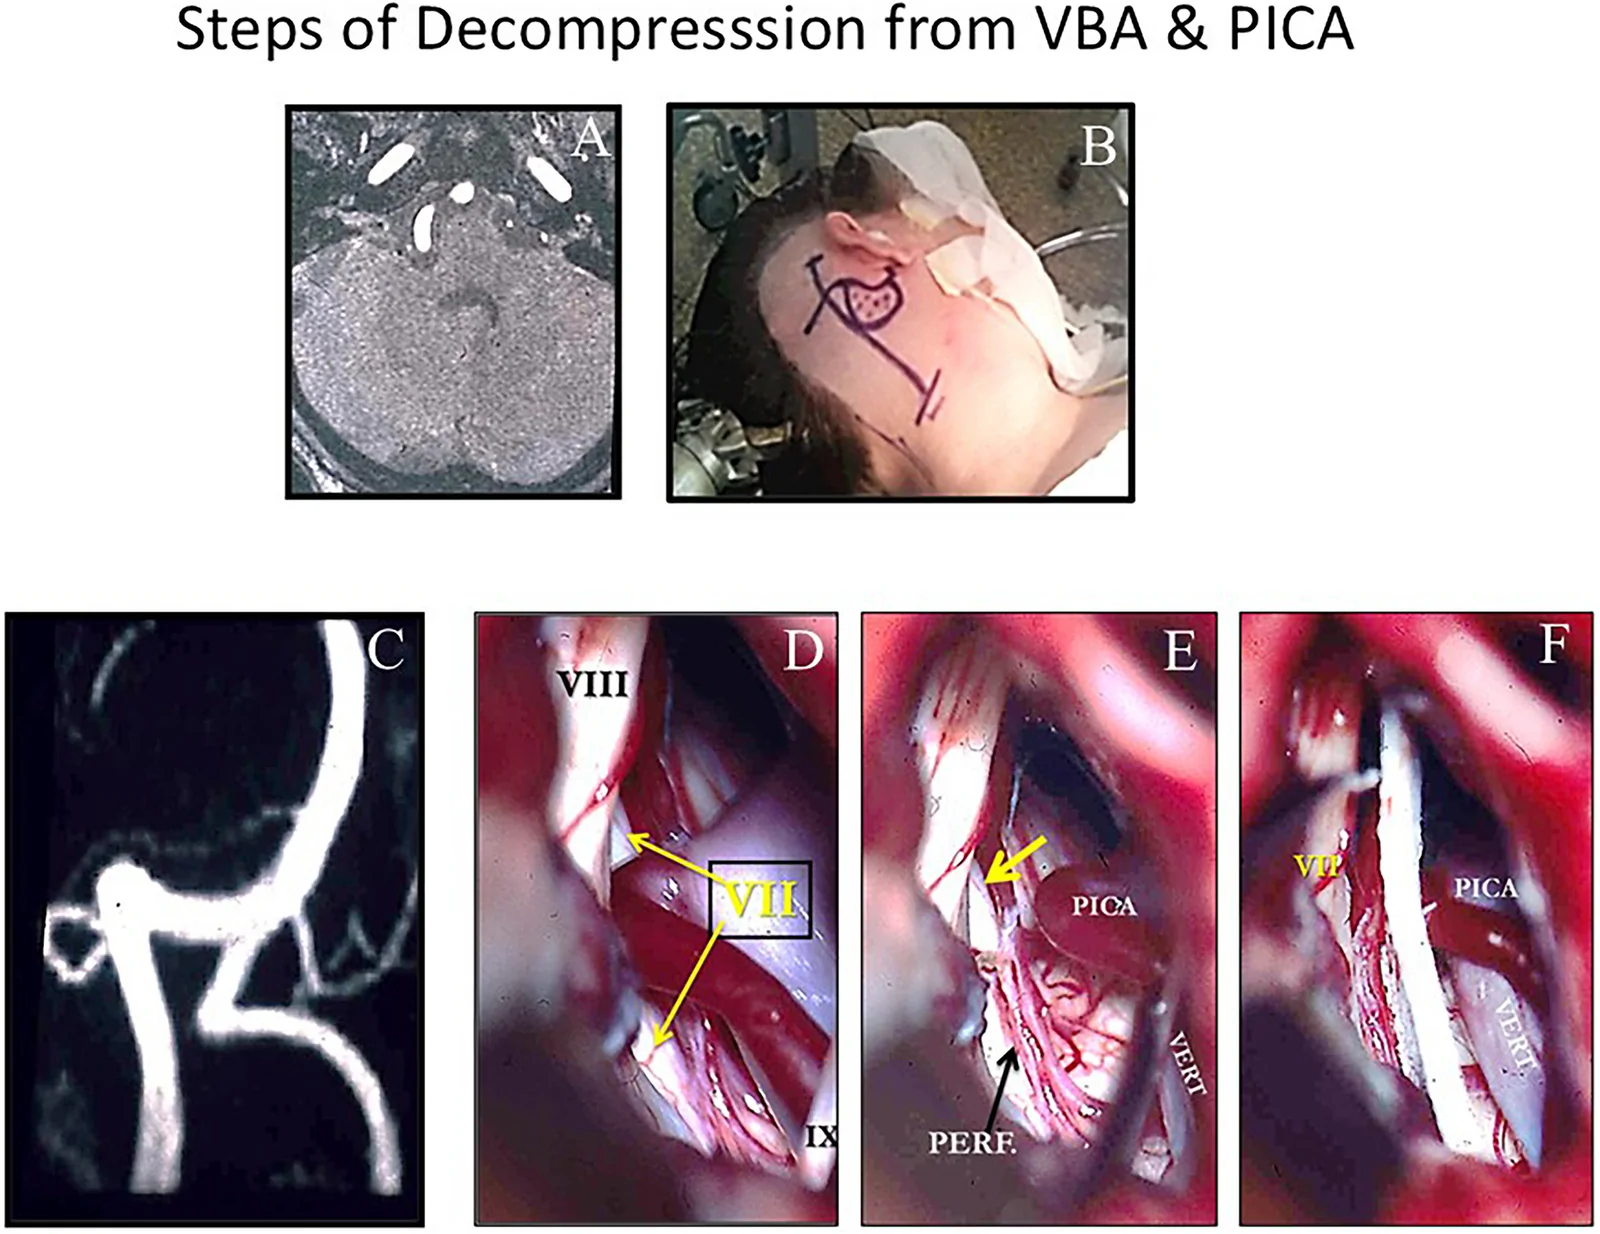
Steps of decompression from Posterior Inferior Cerebellar Artery (PICA) and Vertebro Basilar Artery (VBA). Neurovascular conflict by PICA. A Axial MRI showing VBA and dolichoectatic-PICA conflicting on the right side. B Landmarks of skin incision and craniectomy. C Angio-TOF reconstruction of VBA and PICA. D Microsurgical view of the conflict at the facial REZ (ventrally to the VIIIth CN). E mobilization of PICA. Note the perforators to brainstem (PERF). F PICA and VBA maintained apart by (semirigid) Teflon plate
